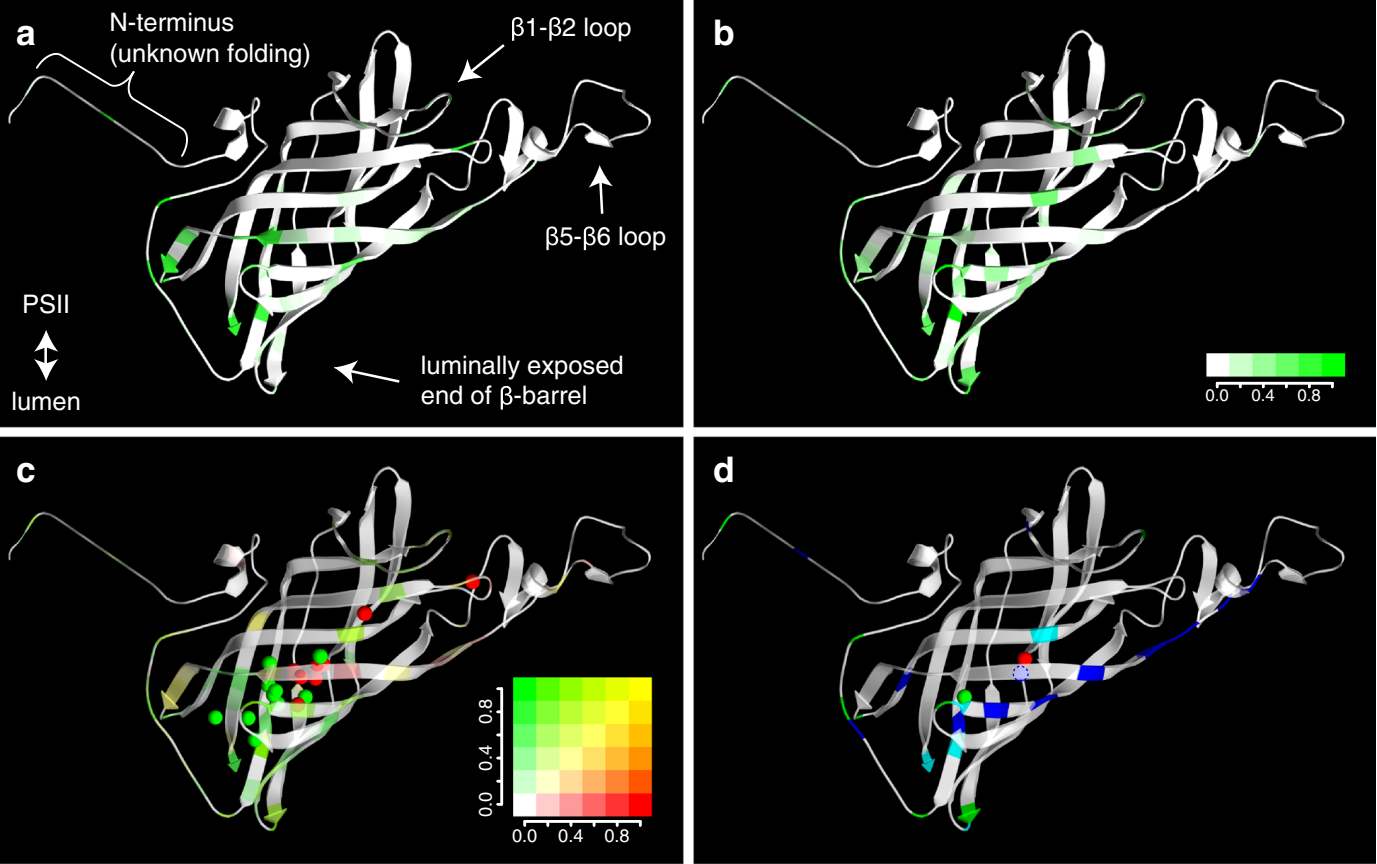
Mapping variable amino acid residues on the PsbO structure. a Differences between isoforms in Solanaceae species and (b) differences between isoforms averaged across all angiosperm families. The varying positions are green-coloured depending on frequency of differences among the analysed pairs of isoforms. c Merged differences between isoforms (in green) and between species (in red) with equally coloured spheres indicating spatial centres of these differences calculated separately for each angiosperm family, the frequency of particular differences on each position is indicated by colour gradient. d Merged averaged differences between isoforms (in green), between plant families (in blue) or both types (in cyan); only positions with a value of variability above a given threshold (0.24) are shown together with overall spatial centres of differences between isoforms, species (within families) and families (green, red and blue spheres, respectively). The homology model of the *Solanum tuberosum* PsbO2 based on the X-ray structure of cyanobacterial PsbO [PDB:3ARC] [5] was constructed using Swiss-Model program [38]; the first 13 N-terminal amino acids were not present in the template structure, so they were pasted in the model without attempts to show any folding and they were not included in calculation of the spatial centres
